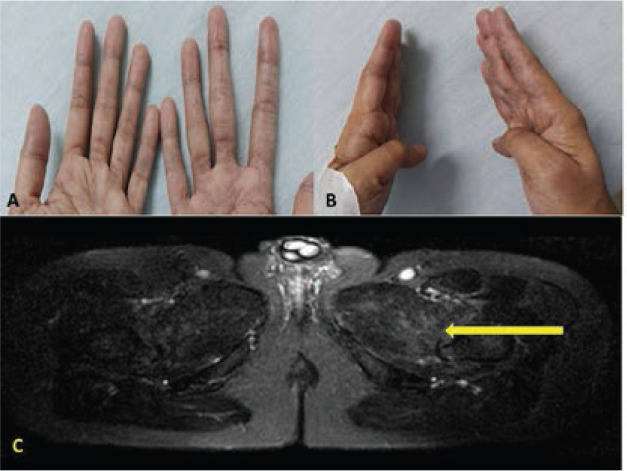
(a)(b) The rough and cracked skin over the radial aspect of the index finger with hyperkeratotic lesion and desquamation: Mechanic’s Hands. (c) MRI axial T2/short tau inversion recovery: The yellow arrow shows the hyperintense signal in the gluteus minimus, iliopsoas, pectineus, and obturator externus consistent with oedema.
